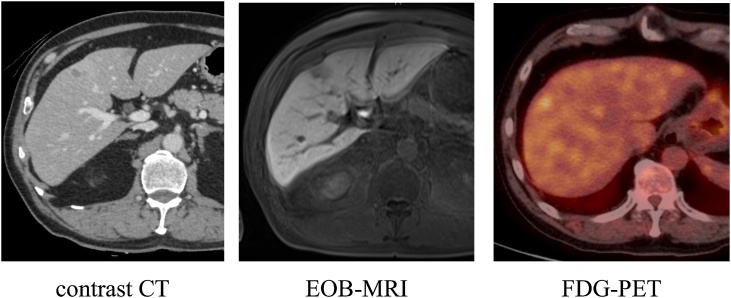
Images of the liver. A: A 10 mm area of low density is observed on the surface in segment 8 of the liver in contrast CT. B: EOB-MRI shows a decrease in uptake of EOB. C: Abnormal FDG accumulation of SUV max 2.8 is seen on PET examination. CT: computer tomography. EOB MRI: gadoxetic acid magnetic resonance imaging. FDG: fluoro-deoxyglucose. SUV: standardized uptake value. PET: positron emission tomography.
